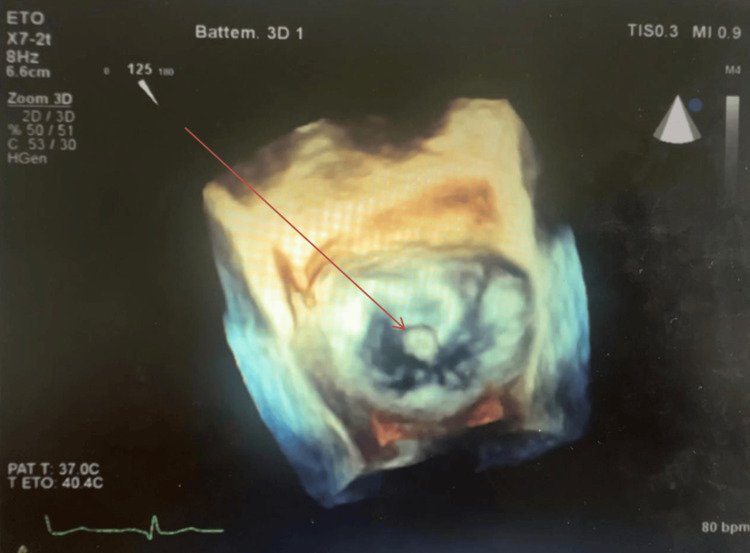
Three-dimensional echocardiography showing the location of the mass on segment A2 of the mitral valve (arrow).
